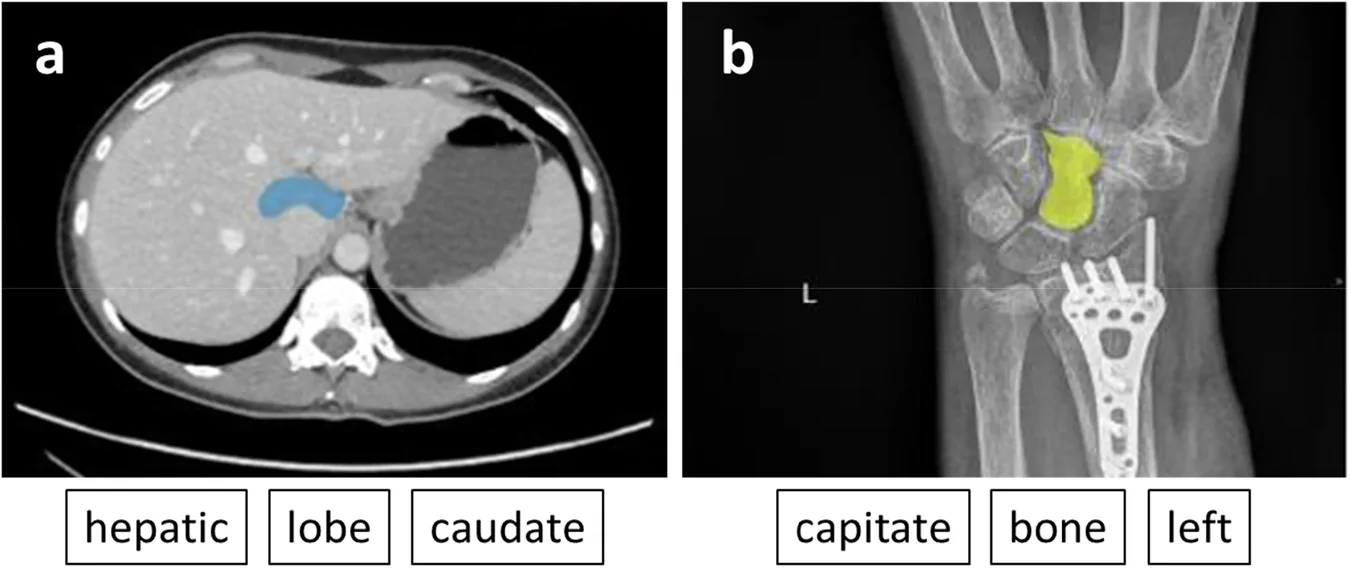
Example images with colored overlaid areas to label the hepatic caudate lobe on an axial CT image (a) and the capitate bone on a wrist conventional X-ray (b)
